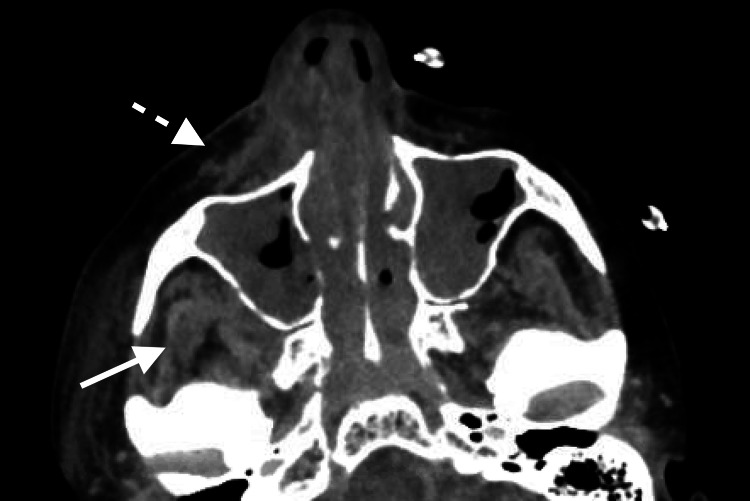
Pre-operative axial CT sinus After presenting with bilateral facial swelling, right maxillary tenderness, and V2 numbness, the patient was found to have fat stranding, thickening, and enhancement of the retroantral (thick arrow) and premaxillary (dashed arrow) spaces on the right, indicative of pseudomonal spread outside of the sinonasal cavity.
